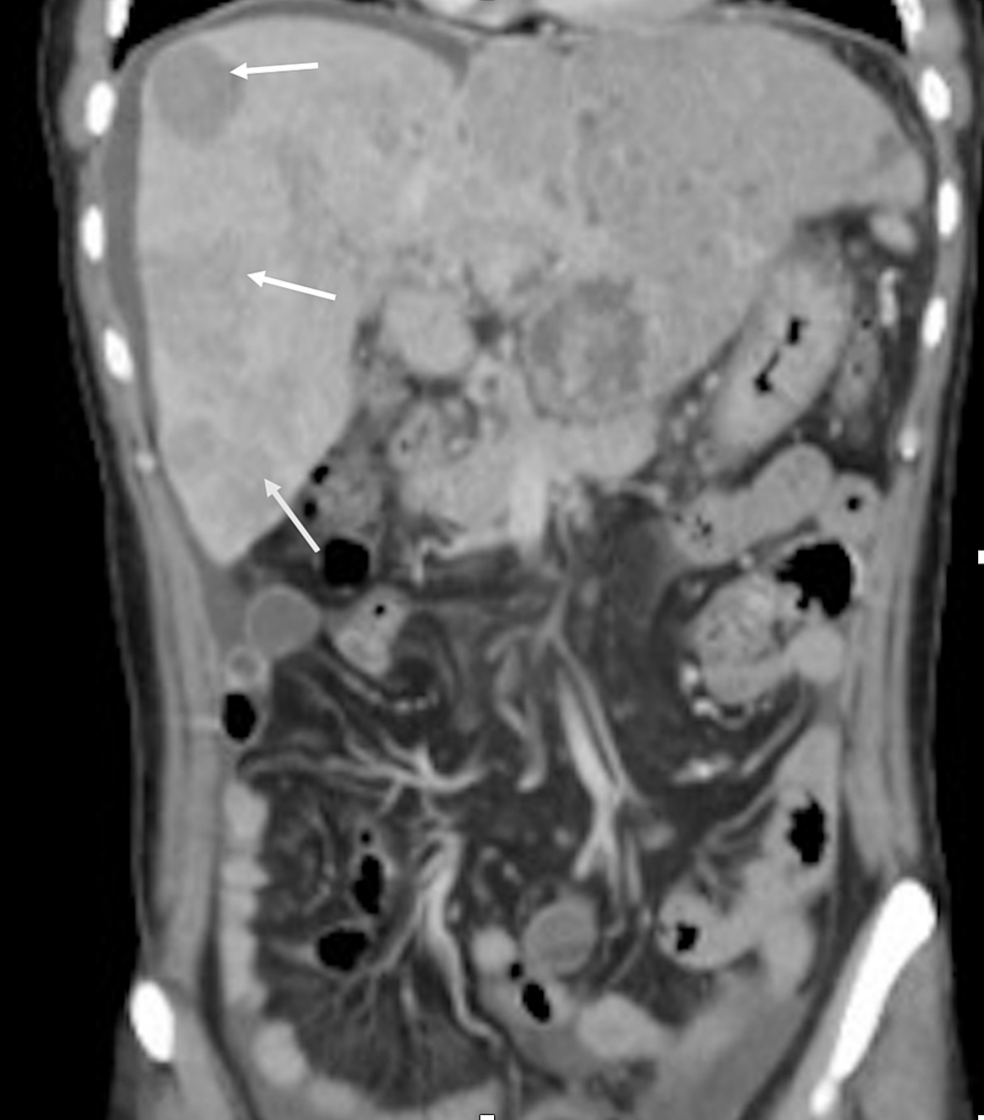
Abdomen CT scan: coronal view White arrow: Multiple hypervascular tumors measuring up to 6 cm in size
